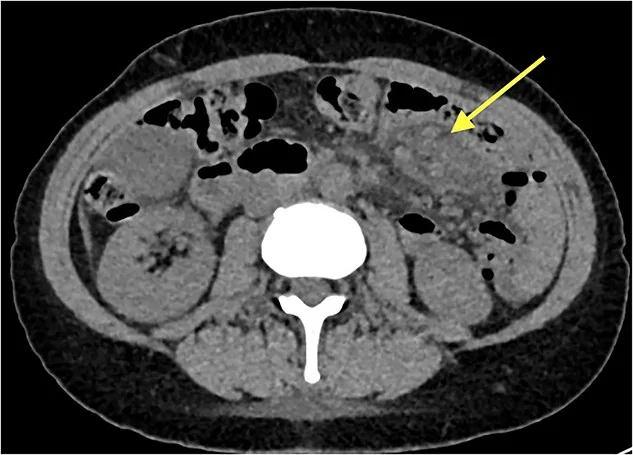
Initial portal venous phase contrast-enhanced CT of the abdomen on Day 1 demonstrating an indeterminate left mid-abdominal lesion (arrow), measuring ~39 mm in diameter, composed of mixed air and soft tissue attenuation. Associated small mesenteric lymph nodes are present. No free intraperitoneal air, free fluid, bowel obstruction, or radiological features of pancreatitis was identified. The differential diagnosis included an infected small bowel diverticulum and interloop abscess.
